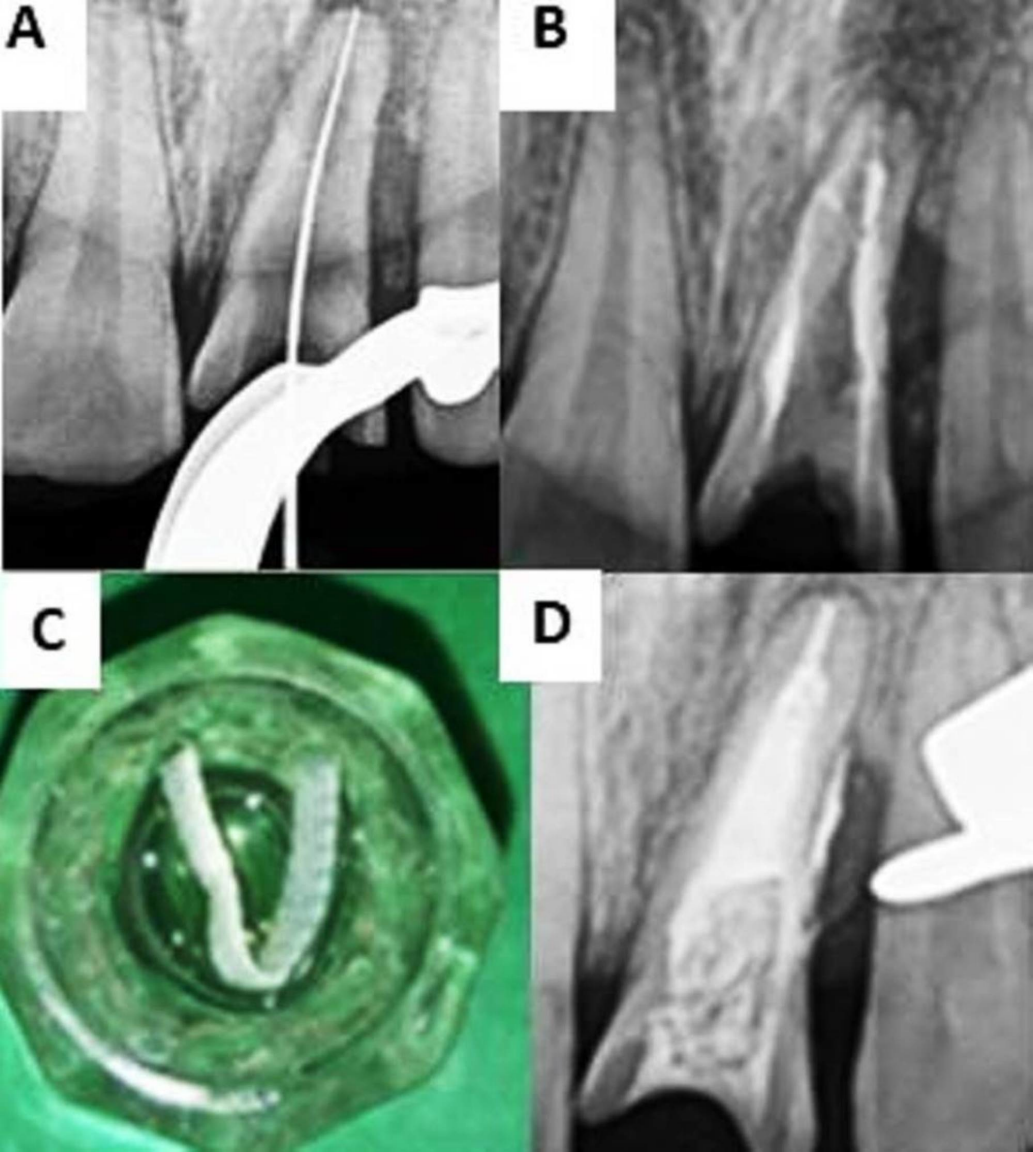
Treatment procedure for tooth 21. (A) Working length determination. (B) Repair of perforation internally using MTA. (C) Ribbond fibres. (D) Post-obturation radiograph. MTA: Mineral trioxide aggregate
